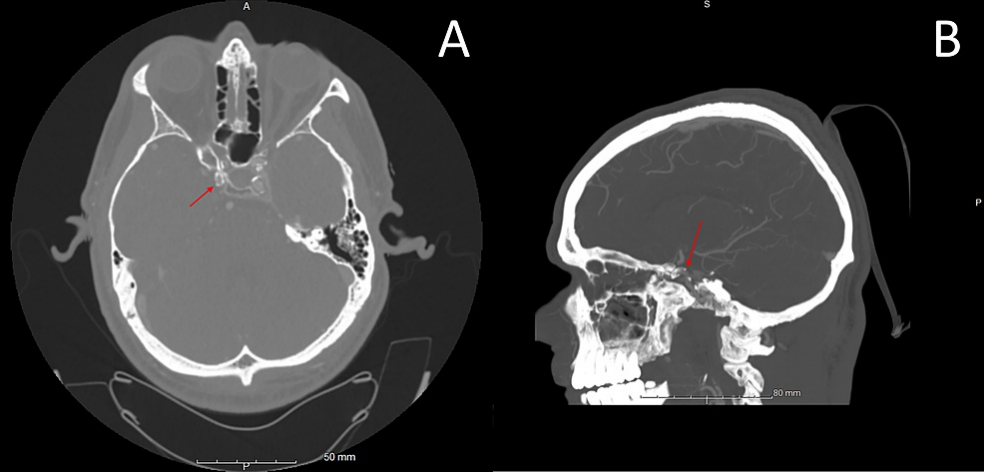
CT angiogram of the head, axial cut (A) and sagittal cut (B) demonstrating atherosclerotic changes in the right clinoid ICA (red arrow) resulting in approximately 70% stenosis per WASID criteria. CT: computed tomography; ICA: internal carotid artery; WASID: Warfarin-Aspirin Symptomatic Intracranial Disease
